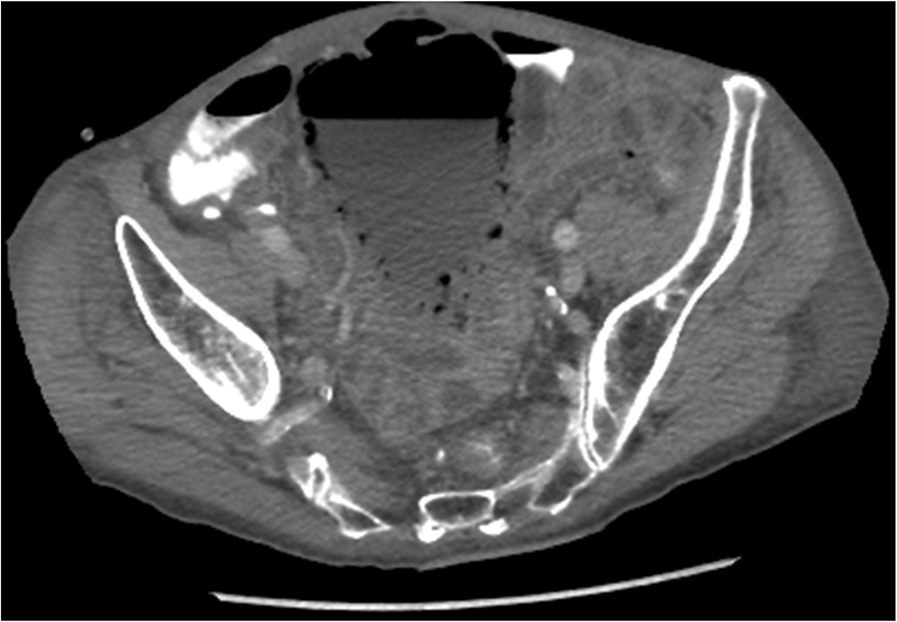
Computed tomography. Axial view showing multiple gas foci in a diffuse collection of gas within the thickened bladder wall.
